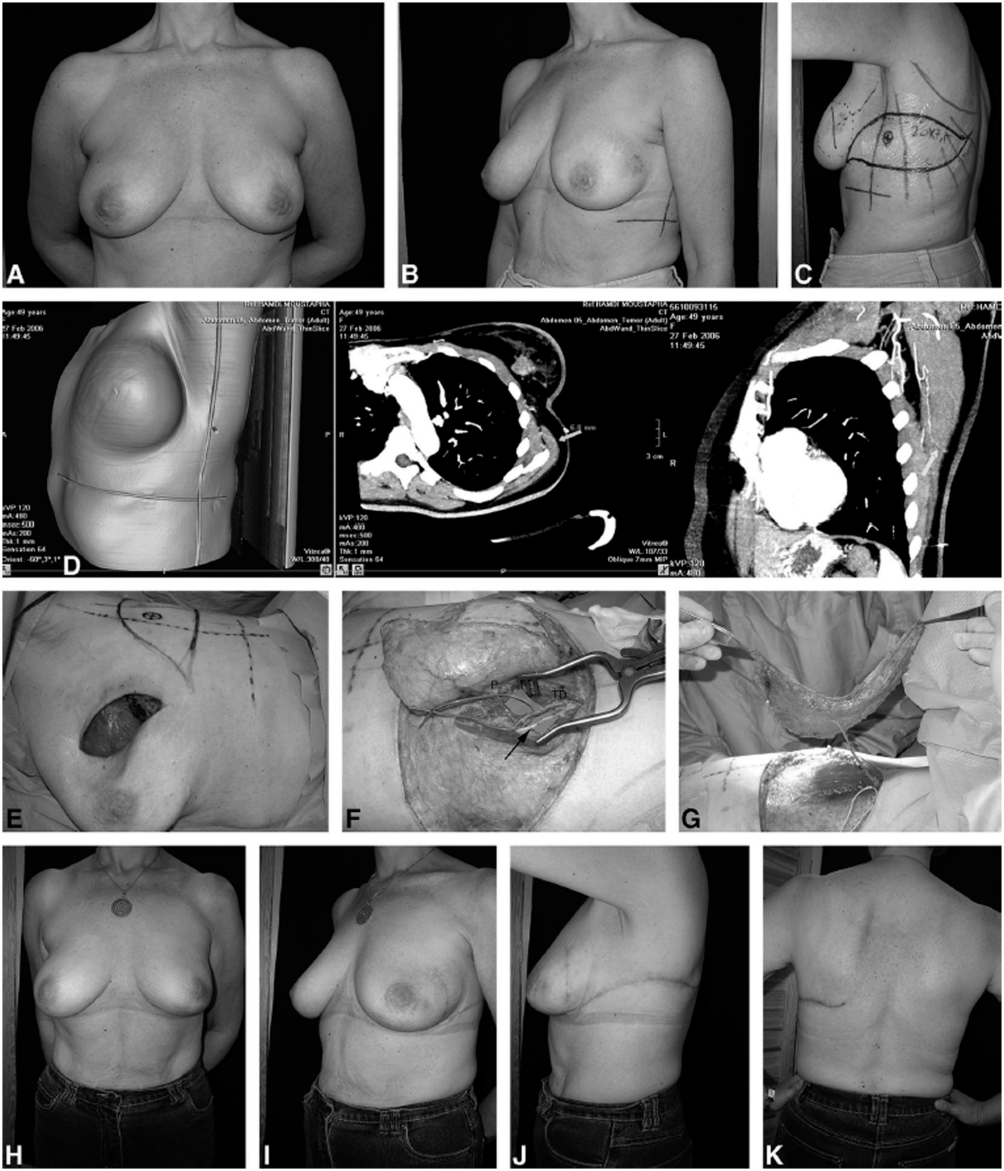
Hamdi et al demonstrated perforator identification on CTA and Volume Rendering (VR) and explained the application onto the patient. (A) A patient having partial breast reconstruction using a pedicled TDAP flap. (A, B) Preoperative views. (C) Marking the TDAP flap and the perforator (circled cross) guided by the CT. (D) CT images with the dominant perforator shown (arrow) on the sagittal and horizontal sections and transferred onto the patient’s 3D image. As a landmark, a lead cross was used on the patient's skin. (E) Resulting breast defect following wide local excision. (F) TDAP perforator dissection up to the thoraco-dorsal vessels (vessel loop) by splitting the LD muscle. Complete preservation of the thoraco-dorsal nerve (arrow). (G) Complete harvest of the TDAP flap. (H, I) Postoperative results at 3 months. (J, K) The donor site. The scar is hidden in the bra region. (used with permission of Wolters Kluwer Health, Inc.).^15^
